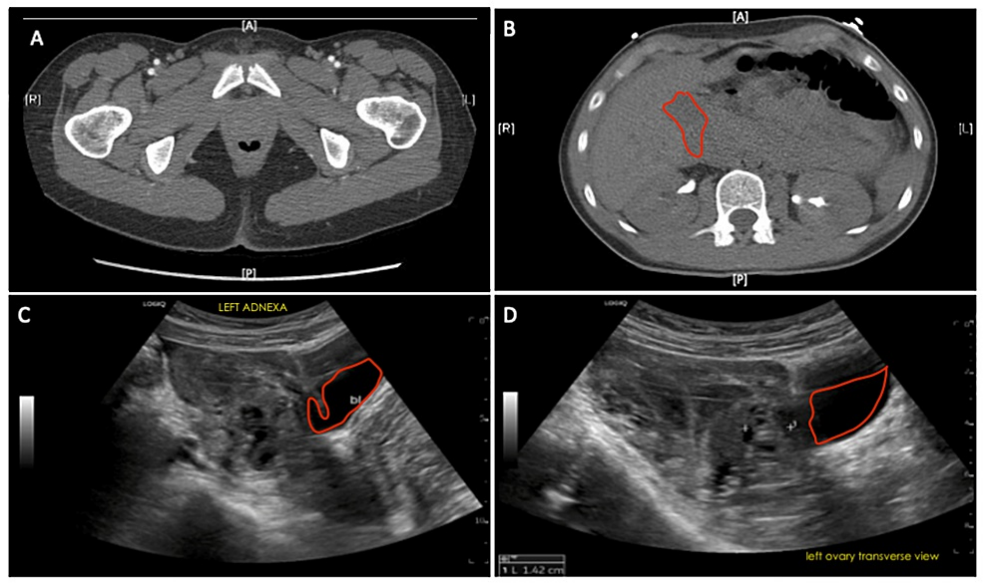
(A, B) CT of the pelvis and abdomen significant for an ill-defined hypodense structure is apparent in the left adnexa, measuring 4.4 x 3.1 cm with high-density fluid layering on top of the uterus and low to intermediate density abdominopelvic free fluid (red outline); hemorrhage is noted throughout the peritoneum and pelvis. (C, D) Non-obstetrical complete pelvic ultrasonography is significant for a complex septated cystic structure in the left ovary/adnexa and a large amount of free fluid (red outlines) and suspected blood in the pelvis, a portion of which appears complex.
